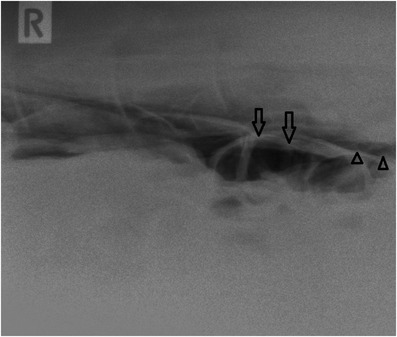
Right latero‐lateral radiograph of the craniodorsal area of the abdomen, image from day of discharge (day 21). Indicating gas‐filled large intestinal loops (arrows) surrounded by small amount of free air in the abdominal cavity (arrowheads)
